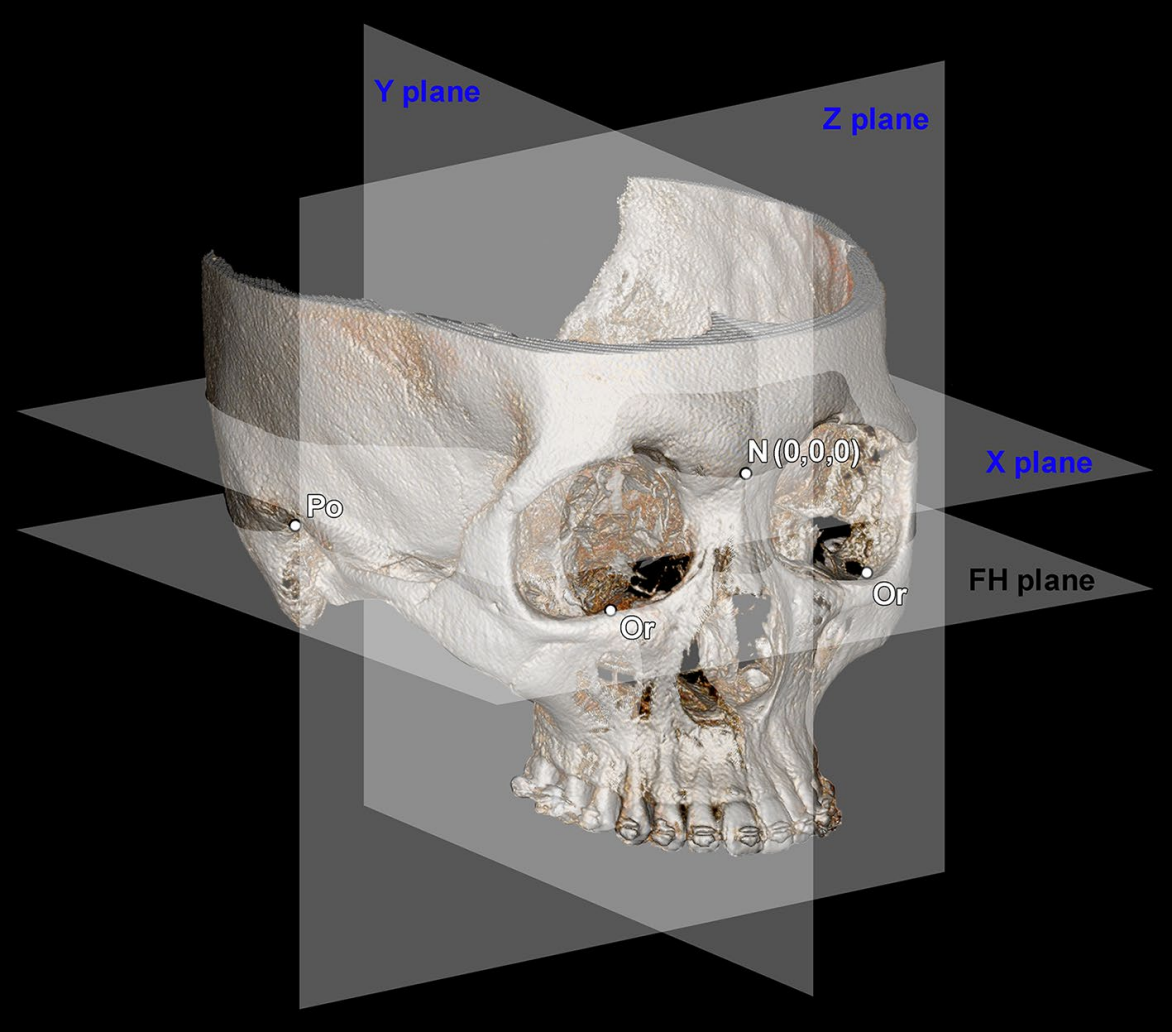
The DICOM data from CBCT were converted to stereolithography format, oriented, and reconstructed following reference planes. The X plane is the plane passing the nasion (N), which is parallel to the Frankfort horizontal (FH) plane passing through the left and right orbitales (Or) and the right porion (Po). The Y plane is the plane passing through the N and basion, while perpendicular to the X plane, and the Z plane is perpendicular to the X- and Y-planes, setting the plane through the N (0, 0, 0).
